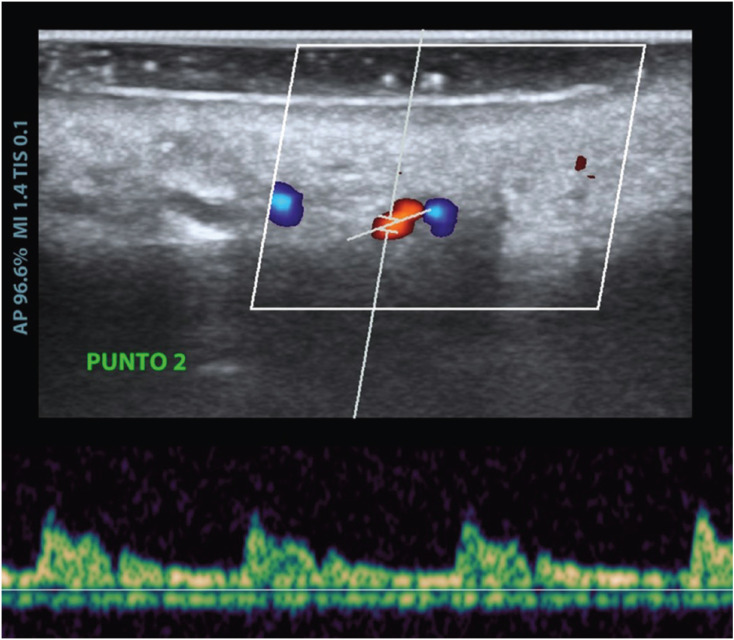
Velocimetry curve and doppler effect (red and blue).
